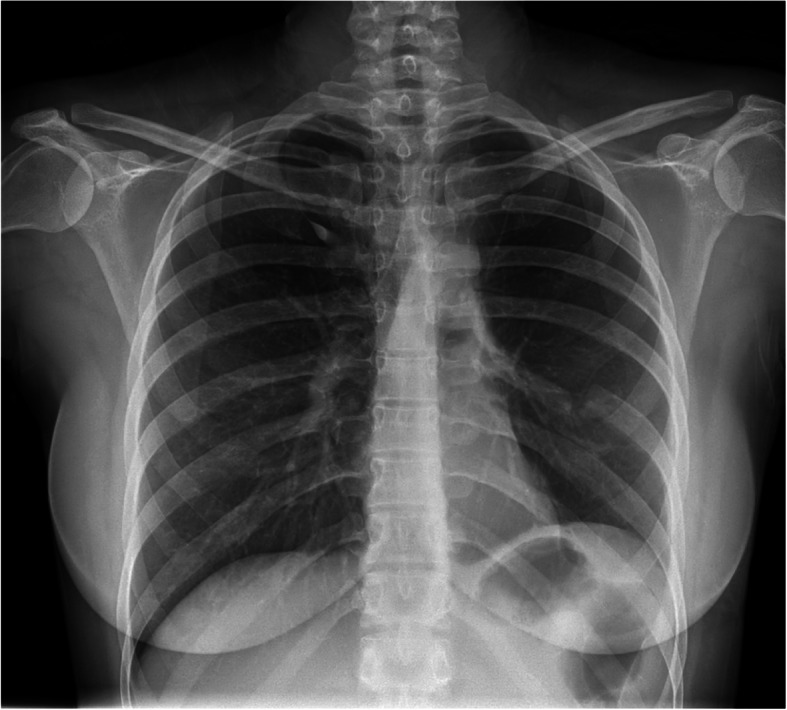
Follow-up chest x-ray of a 27-year-old female 4 month after left upper lobe lobectomy
